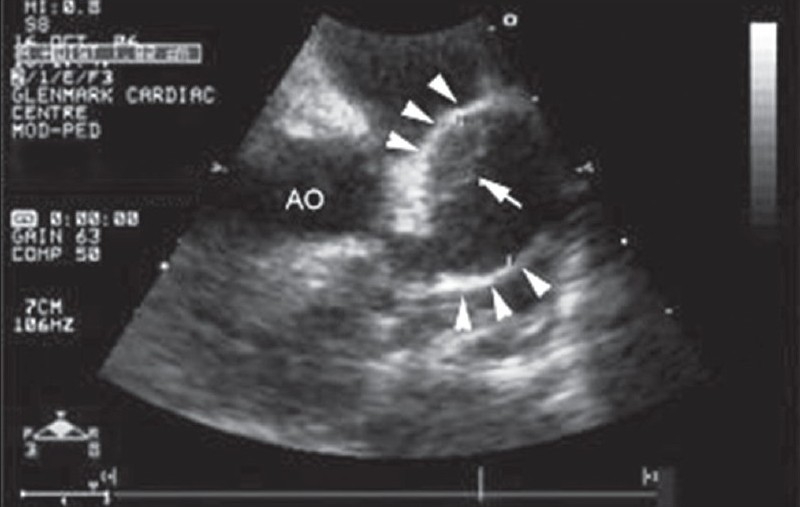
Two-dimensional echocardiography in short-axis view at the level of aortic valve showed a large aneurysm (arrow heads; 18 mm) involving the proximal left anterior descending artery (LAD) with the presence of thrombus (arrow)
